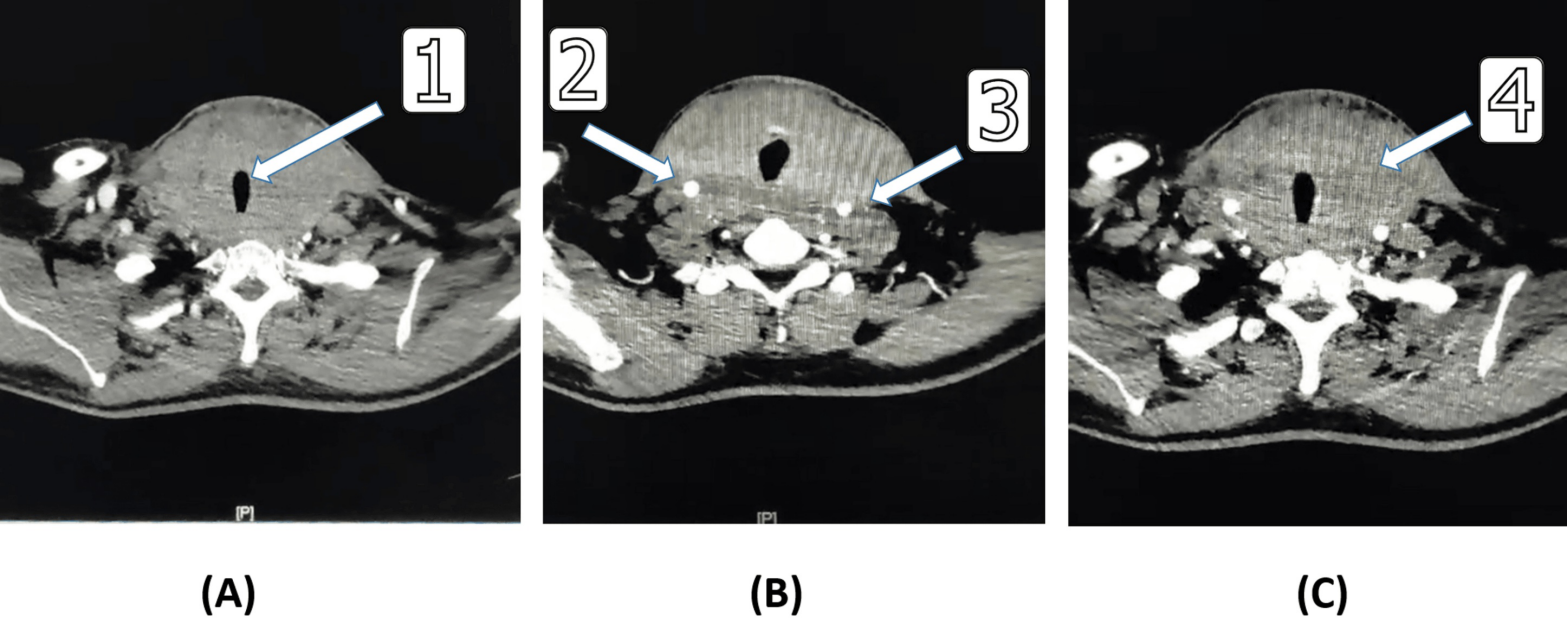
Post-contrast CT neck Arrow 1: Severe tracheal compression resulting in tracheomalacia. Arrow 2: Complete encasement of the carotid sheath, involving both the carotid artery and internal jugular vein. Arrow 3: Significant posterior compression of the esophagus, leading to dysphagia. Arrow 4: Marked retrosternal extension of the thyroid gland, more pronounced on the left side.
